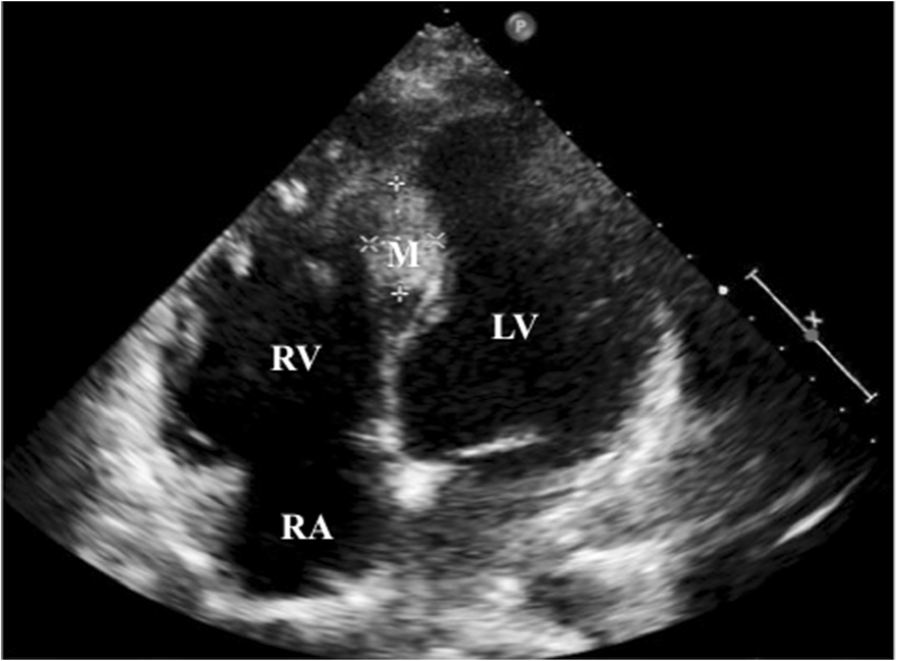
Transthoracic echocardiography image showing a mass in the intraventricular septum. *RA* right atrium, *RV* right ventricle, *LV* left ventricle, *M* mass
